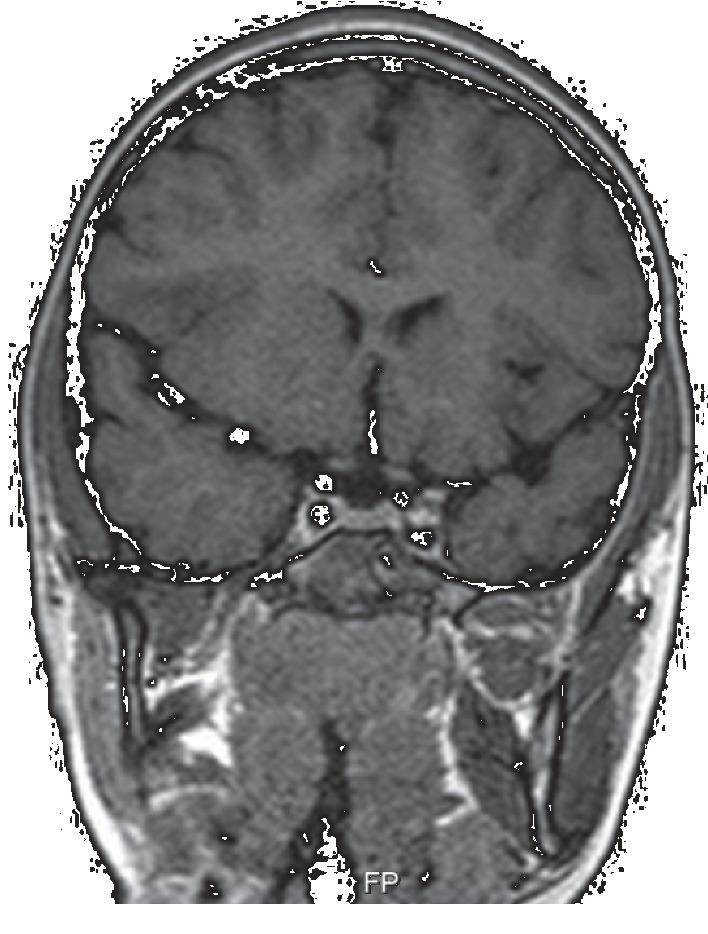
Coronal contrast-enhanced T1-weighted image shows a heterogeneous abnormal enhancement of left cavernous sinus.
